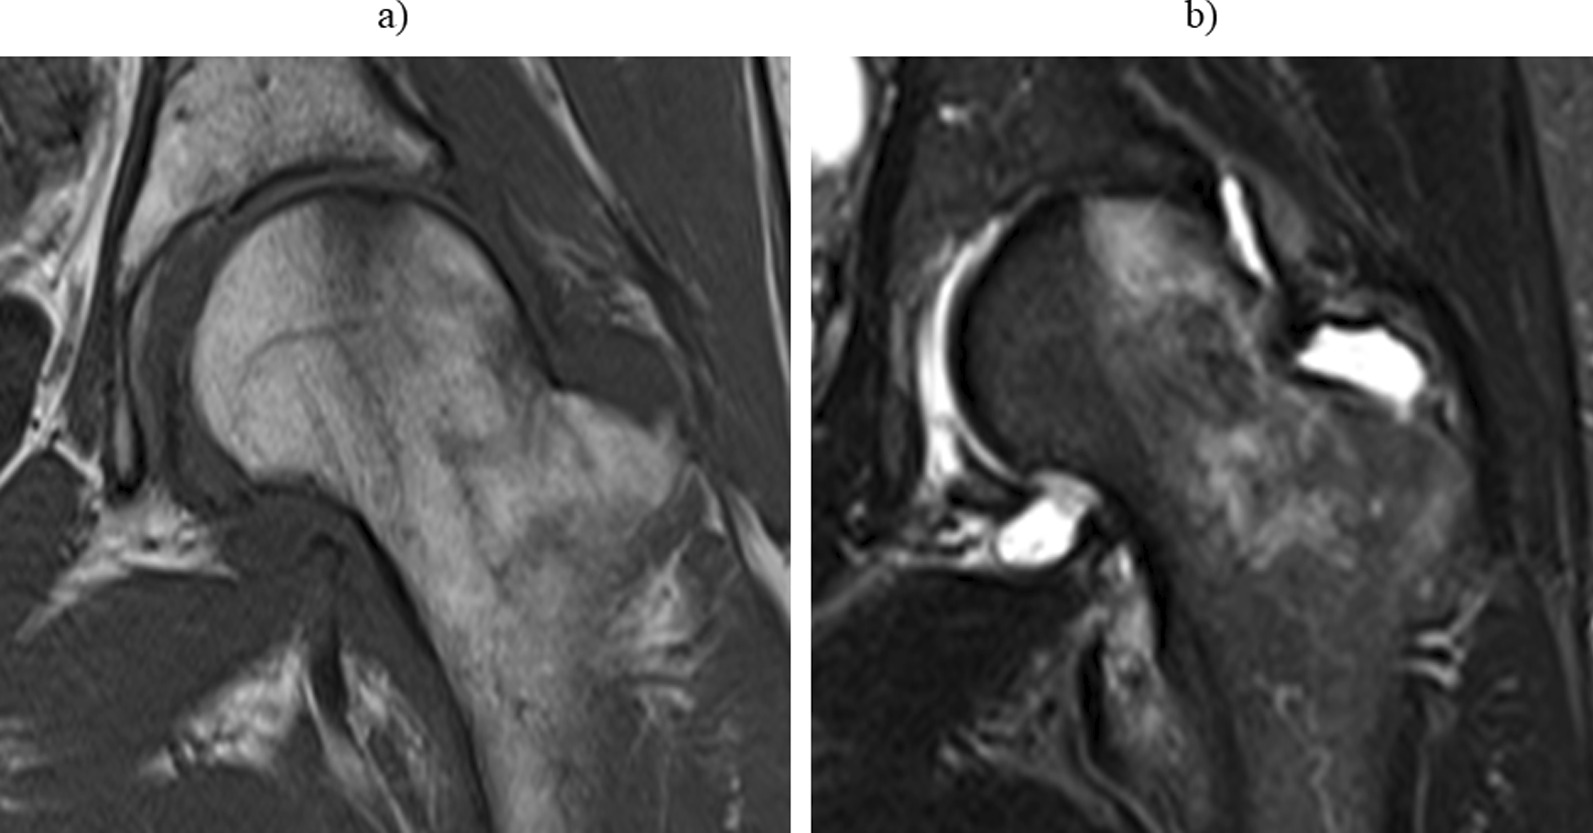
An example of bone marrow oedema (BME) in the femoral head. Magnetic resonance images of a middle-aged woman who was imaged 2 months after left hip pain without an apparent trigger. BME shows low signal intensity on a coronal T1-weighted image (a) and high signal intensity on a coronal short tau inversion recovery image (b). On measurement, the size of the BME was 3.2 cm. *BME* Bone marrow oedema
